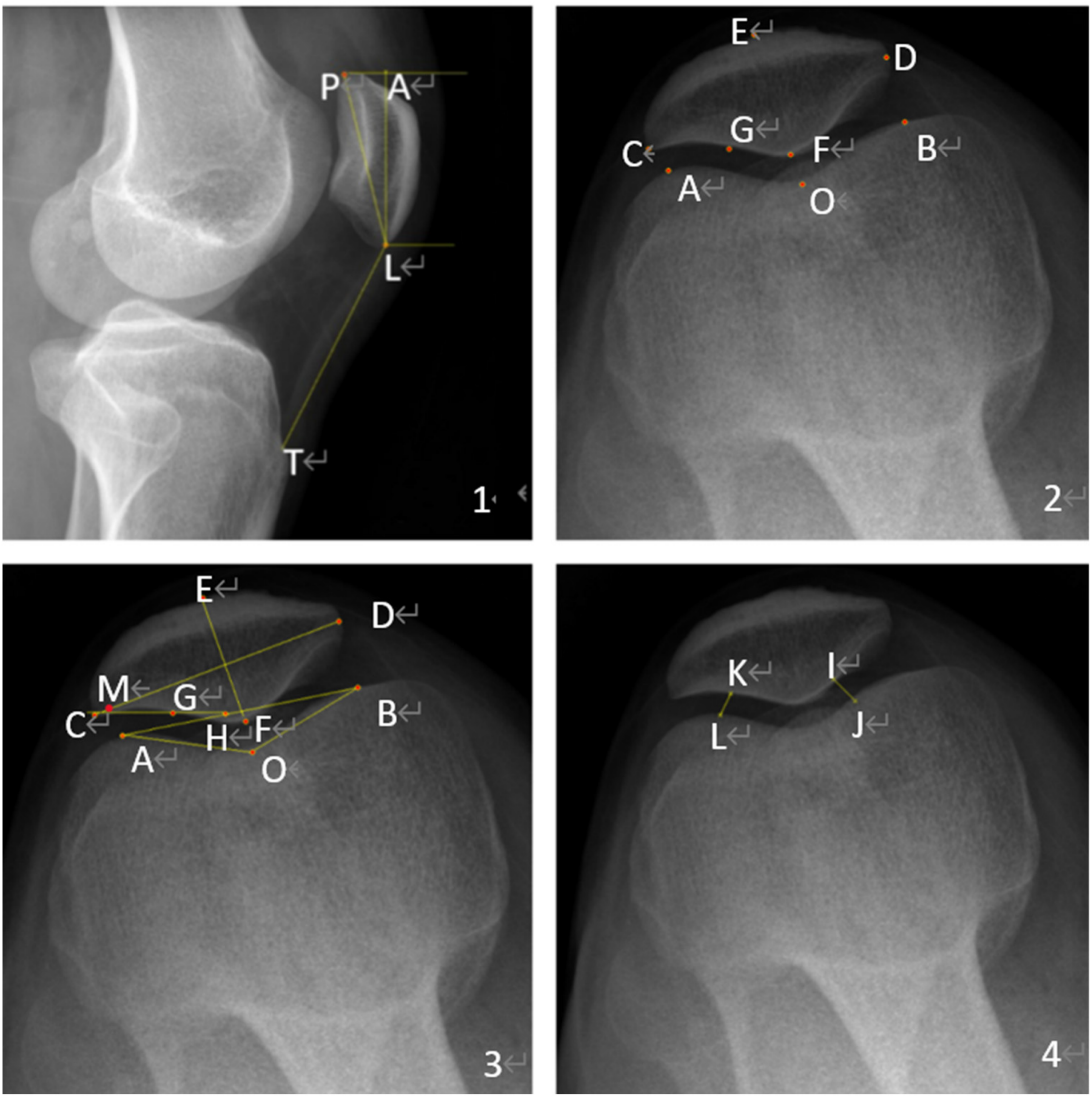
Measurement parameters for the patellofemoral. 1. X-ray of the lateral patellofemoral. 2. Location of landmarks on an axial x-ray image. P superior pole of the patella. L Inferior pole of patella. T Tibial tuberosity inferior pole. The Insall-Salvati ratio = LT/LP, and the patellar length = AL (Figure 2.1). The highest point of the lateral condyle. B Highest point of the medial condyle. C Outermost point of patella. D Most medial point of patella. E Anterior cortex of the patella. F Median cartilaginous ridge of patella. G Tangent point of the lateral articular surface of the patella (Figure 2.2). H The intersection of the lateral patella's articular surface and the femoral internal and external condyles line. M is the point at which the highest point of the lateral epicondyle of the femur intersects with the width of the patella. O Most distal point at the bottom of the trochlear groove Patellar width = CD, Patella thickness = EF. Groove angle = angle AOB. Patellar displacement rate = CM/CD. Lateral patellofemoral angle = angle GHA (Figure 2.3). IJ, KL, the patellofemoral joint's internal and lateral spaces, are connected in the shortest distance. Patellofemoral index = IJ/KL (Figure 2.4).
